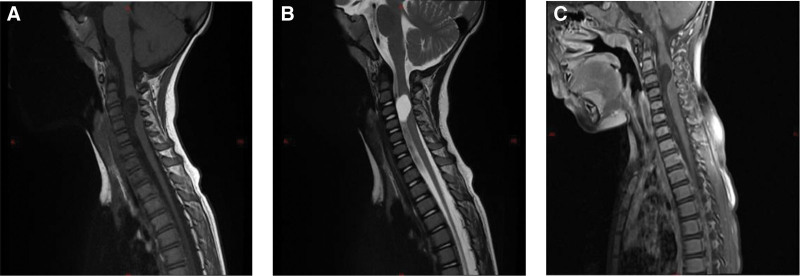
Preoperative magnetic resonance imaging imaging findings. (A) The sagittal T1-weighted image shows a hypointense lesion on the ventral side of the spinal canal at the cervical vertebra2–cervical vertebra 4 segments. The spinal cord is compressed and displaced posteriorly. (B) The sagittal T2-weighted imageshows that the lesion is hyperintense, and the dorsal side of the spinal cord is compressed and deformed. (C) The sagittal contrast-enhanced T1-weighted image shows that the lesion has no obvious enhancement, and the spinal cord is compressed. T1 = Longitudinal Relaxation Time T2 = Transverse Relaxation Time.
